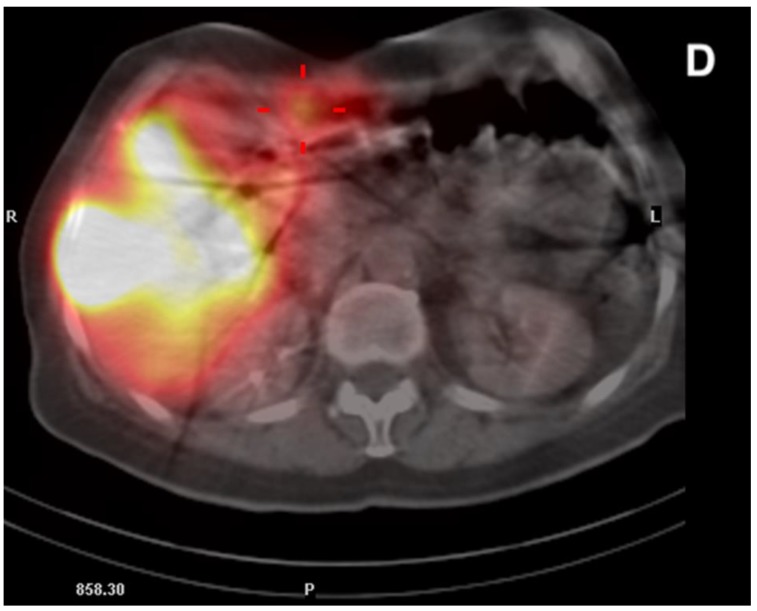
(A) Angiogram of the left hepatic artery showing falciform artery travelling medially beyond the margin of the liver. (B) Its presence was confirmed on Dyna-CT and showed contrast enhancement of the abdominal wall (arrows) (C), and on SPECT/CT after ^99m^Tc-MAA injection (D) (*crosshairs*).
